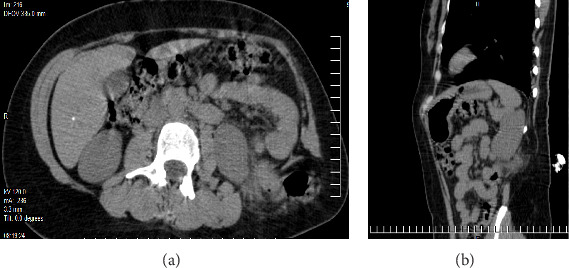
(a, b) A simple and contrast-enhanced abdominal computed tomography scan revealed an aponeurotic defect located in the left lumbar region within the Grynfeltt–Lesshaft tract, measuring 69 × 51 mm, with a hernial sac containing retroperitoneal fat.
